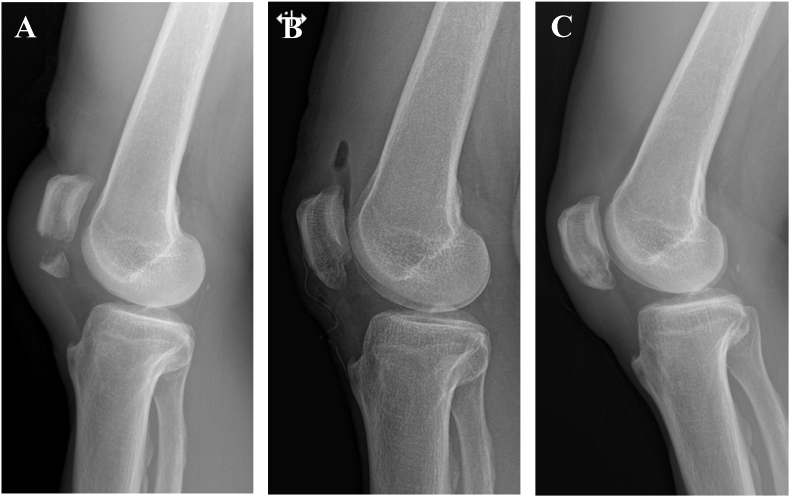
A 55-year-old man underwent suture anchor fixation for a left patellar inferior pole fracture. (A) Preoperative knee radiograph (B) Immediate postoperative knee radiograph (C) 24 months postoperatively.
